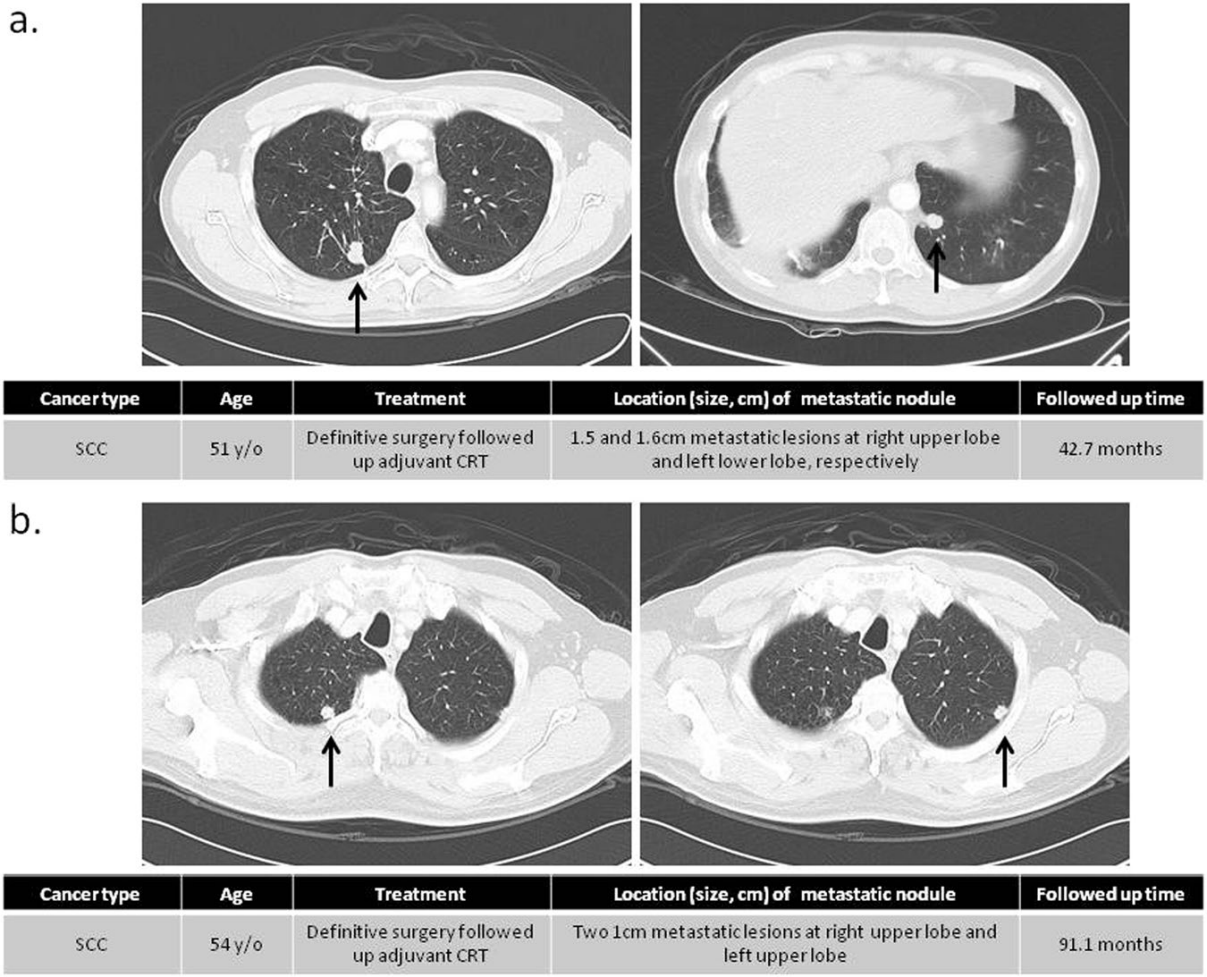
Clinical information and computed tomographies of pulmonary metastases. (a) Oligometastases of the bilateral lung were found until month 47.1 following diagnosis, including metastases to the right upper lobe (RUL) and left lower lobe (LLL) of the lung. (b) Two subpleural metastatic nodules approximately 1 cm in diameter were found in bilateral apices of the lung 42.4 months after the initial diagnosis. There were no other significant metastatic lesions in the distal lymph nodes, bone, or bilateral adrenal glands.
